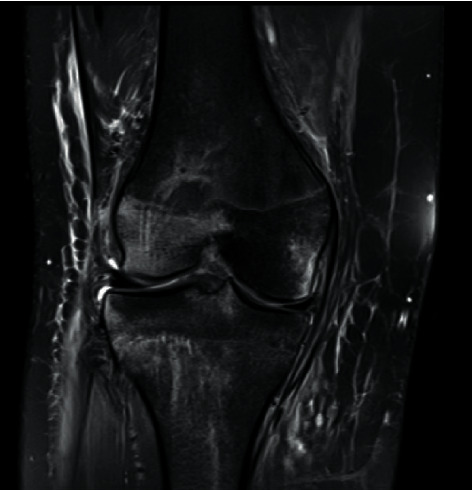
The patient also underwent magnetic resonance imaging (MRI) of the knee, which confirmed the distal patellar tendon avulsion, and demonstrated a complete tear of the anterior cruciate ligament (ACL), associated bone bruises on the lateral femoral condyle, and also on the posterolateral tibial plateau. The posterior cruciate ligament and the MCL were undamaged.
